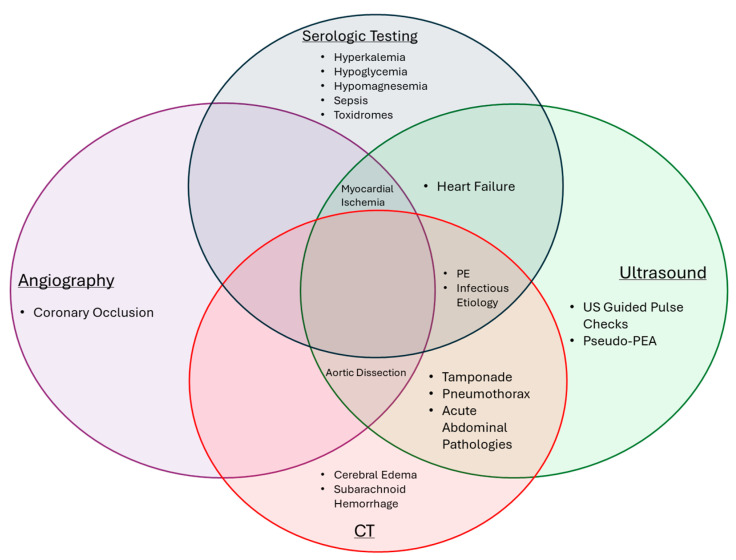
Diagnoses made possible by various investigational modalities. Investigational modalities are represented by large circles, with the causes of cardiac arrest that they can identify in the area of the circles. CT, computed tomography; PE, pulmonary embolism; US, ultrasonography; and PEA, pulseless electrical activity.
